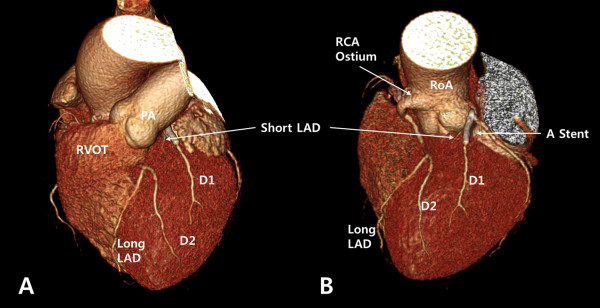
Computed tomographic coronary angiography. (A) LAO cranial view showing the dual LAD anomaly with the short LAD on the proximal AIVG, giving rise to the D1 and the long LAD entering the mid AIVG giving rise to the D2. (B) LAO cranial view with the RVOT removed, showing the short LAD originating from the left main coronary artery and the long LAD originating from the proximal RCA. The proximal RCA is taking off above the RCS and the long LAD is branching from the proximal RCA, passing between the RVOT and the aortic root. A stent can be observed from the proximal portion of the short LAD to the D1. The D2 is the dominant artery in the anterior wall of the left ventricle. LAO, left anterior oblique; LAD, left anterior descending artery; LMCA, left main coronary artery; RCA, right coronary artery; AIVG, anterior interventricular goove; D1, first diagonal artery; D2, second diagonal artery; RVOT, right ventricular outflow tract; PA, pulmonary artery; RoA, root of aorta.
